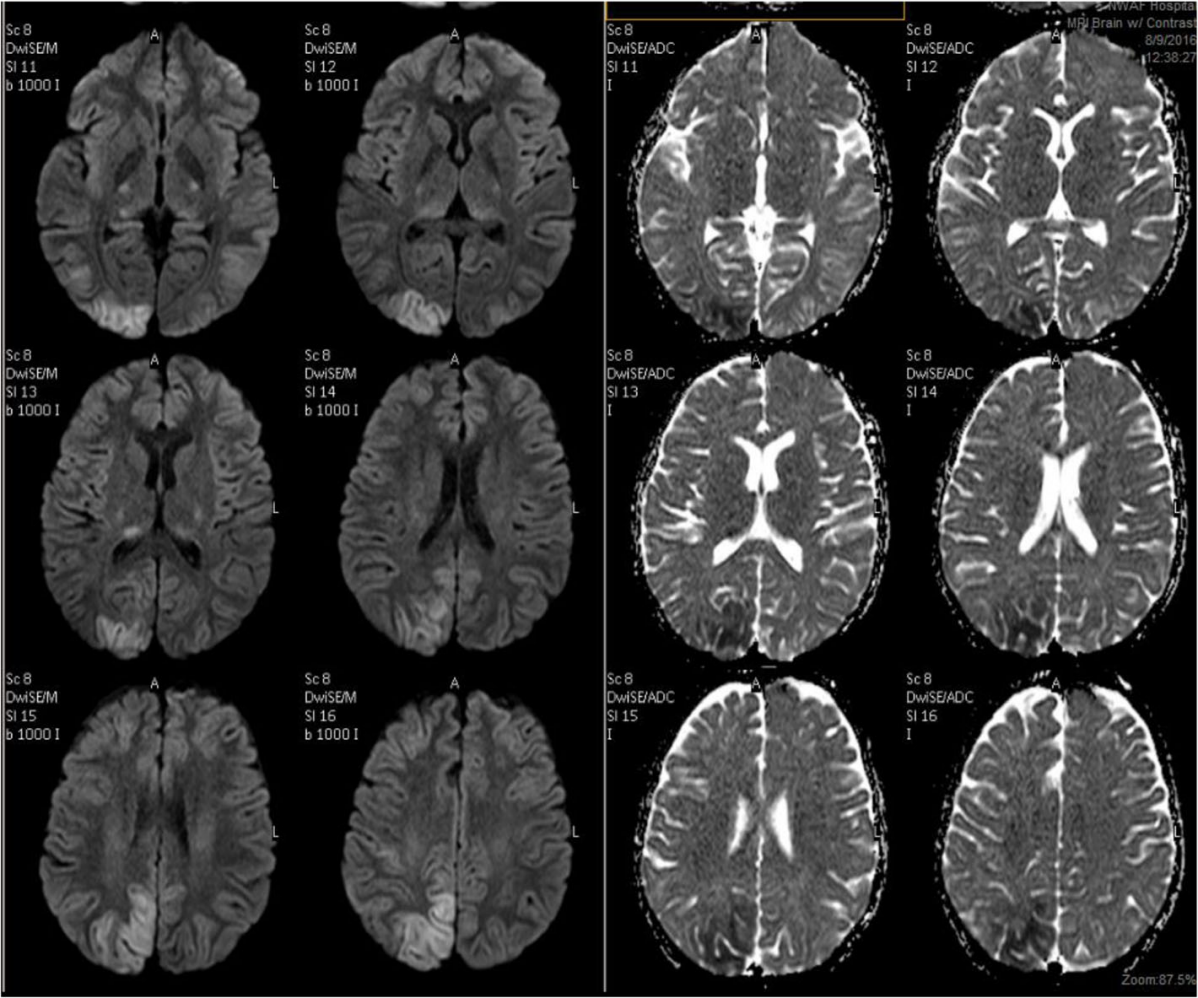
Brain magnetic resonance imaging scans showing tiny areas of recent infarctions with a picture diagnostic of posterior reversible encephalopathy syndrome
